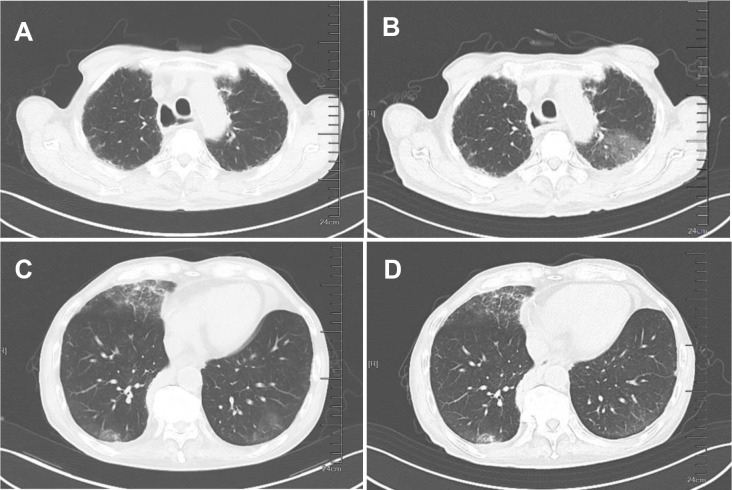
Chest CT images of the patient before and after treatment. (A, C) The patient’s chest CT upon admission on Day 9. (B, D) The patient’s chest CT after anti-inflammatory treatment on Day 14.
